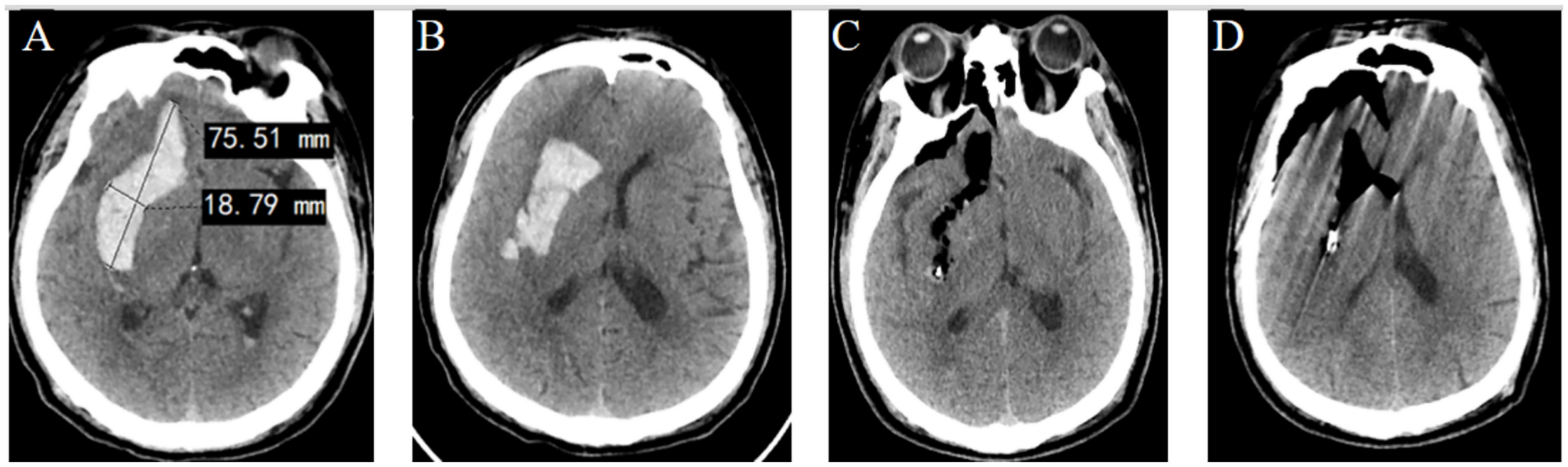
Pre- and post-operation CT scans of an irregular basal ganglia hemorrhage that was evacuated by FATSE combined with a “Two-in-One” neuroendoscopic approach. (A) Pre-operation CT scan showing the irregular “S” shaped hematoma located in the right basal ganglia with an anterior–posterior diameter of approximately 9.7 cm and lateral to medial diameter of 3.5 cm with a width-to-length ratio <50%. (B) The hematoma shaped was irregular. (C) Postoperative CT scan show route of evacuated hematoma was the same of preoperative hematoma shape. (D) Postoperative CT scan showed that the hematoma was almost completely evacuated.
